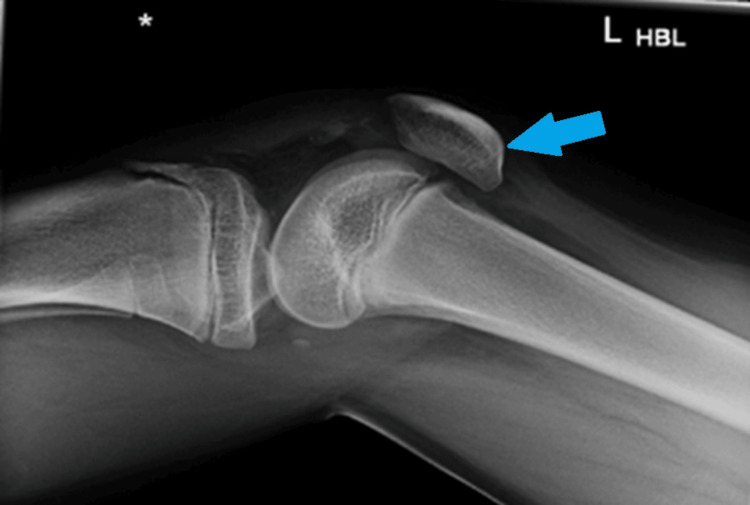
X-ray lateral view on initial presentation. The blue arrow shows patella alta and an abnormal Insall-Salvati ratio.
